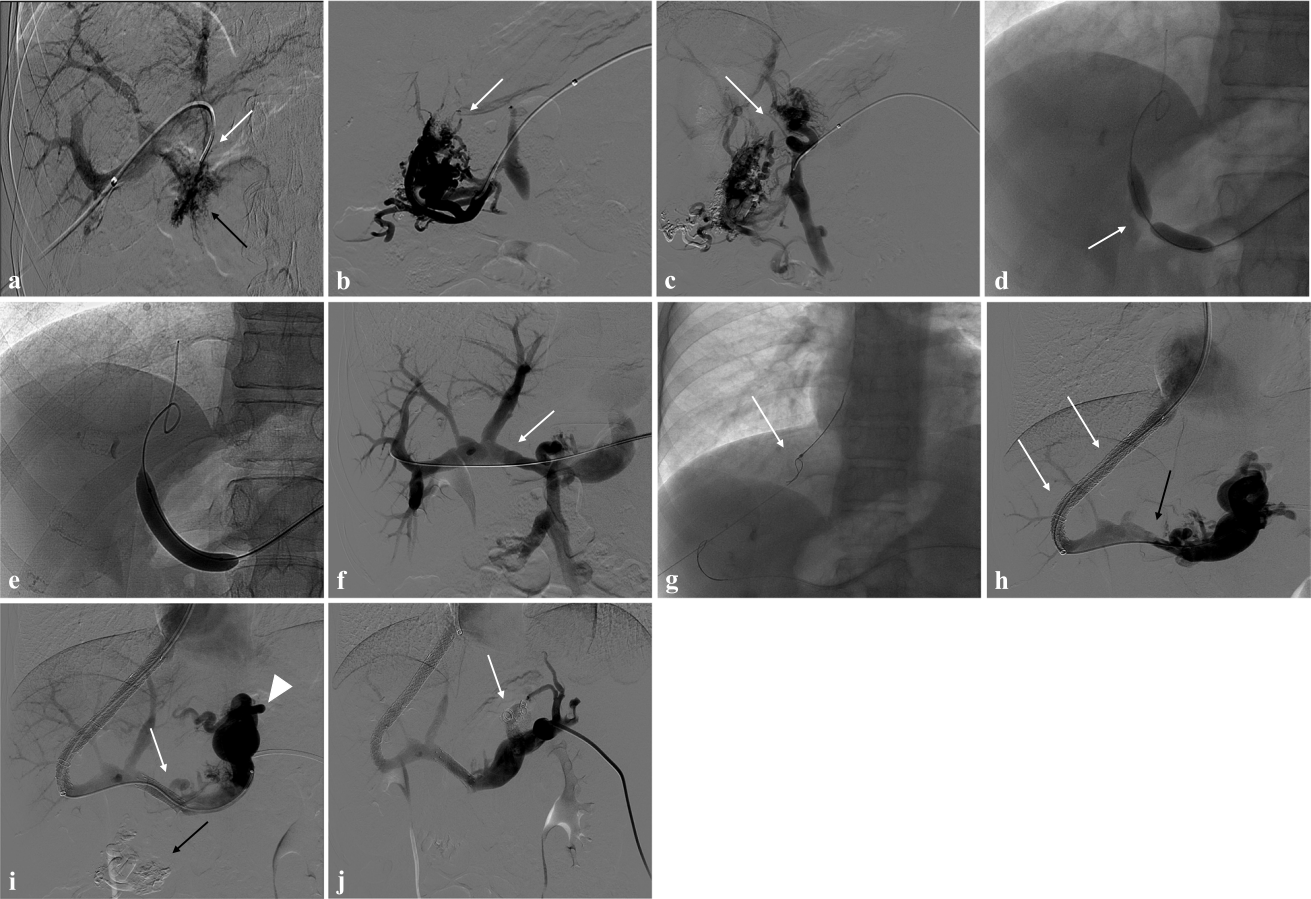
Portal vein CTO in a 21-year-old boy with a history of portal hypertension 9 years after liver transplantation (whole graft) for biliary atresia. a Percutaneous transhepatic portography shows complete extrahepatic portal vein thrombosis (white arrow), with opacification of tiny irregular portal cavernomatous vessels at hepatic hilum (black arrow). b Percutaneous transplenic anterograde portography shows complete extrahepatic portal vein thrombosis and cavernous transformation (arrow). c The tiny vestige of the main portal trunk was detected (arrow) on the transplenic superior mesenteric vein portography. d, e Fluoroscopic image shows angioplasty of the main portal vein, performed through a 12-mm non-compliant balloon catheter. The focal notch (arrow) representing the tight anastomotic stenosis that probably led to secondary thrombosis was resolved after high-pressure inflation of the non-compliant 12-mm balloon catheter. f Control portography shows an expanded main portal vein with improved hepatopetal portal flow, but still with irregular profiles (arrow). g Through a hybrid transhepatic and transjugular approach under combined ultrasound and fluoroscopic guidance using a modified “gun-sight” technique, the connection between the intrahepatic portal vein and the vena cava was created advancing a 0.018″ micro guidewire into the right atrium. The wire was snared through the right transjugular access providing a through-and-through access (arrow) for transjugular intrahepatic portosystemic shunt (TIPS) placement. h Portography image shows the deployment of two imbricated Viatorr stents to create the TIPS (arrows), with regular intrastent opacification. Despite previous angioplasty, a prestenotic aspect of the main portal vein is seen (black arrow). i Portography image shows a self-expandable 9-mm metallic stent placed to cover the main portal vein trunk stenosis (white arrow). Note subtraction artifacts (black arrow) representing the result of variceal embolization using a mixture of N-butyl cyanoacrylate and iodized oil and metallic coils. Large gastric varices were seen (arrowhead). j Control portography shows regular portal vein axis opacification with TIPS patency and preserved opacification of intrahepatic portal branches; gastric varices disappeared after their embolization (arrow)
